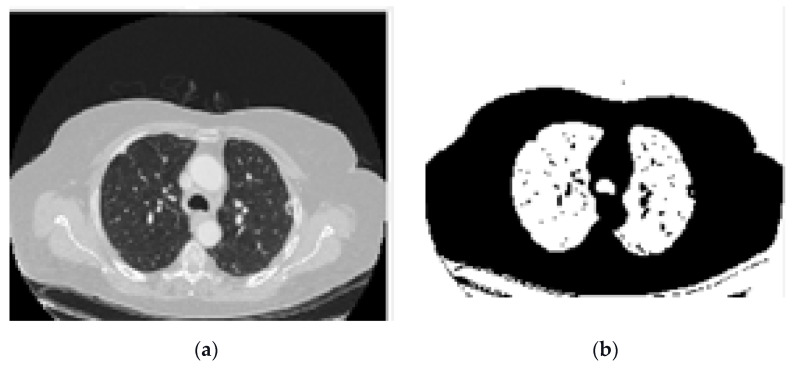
Image binarization process. (a). Original CT, (b). binarized.
